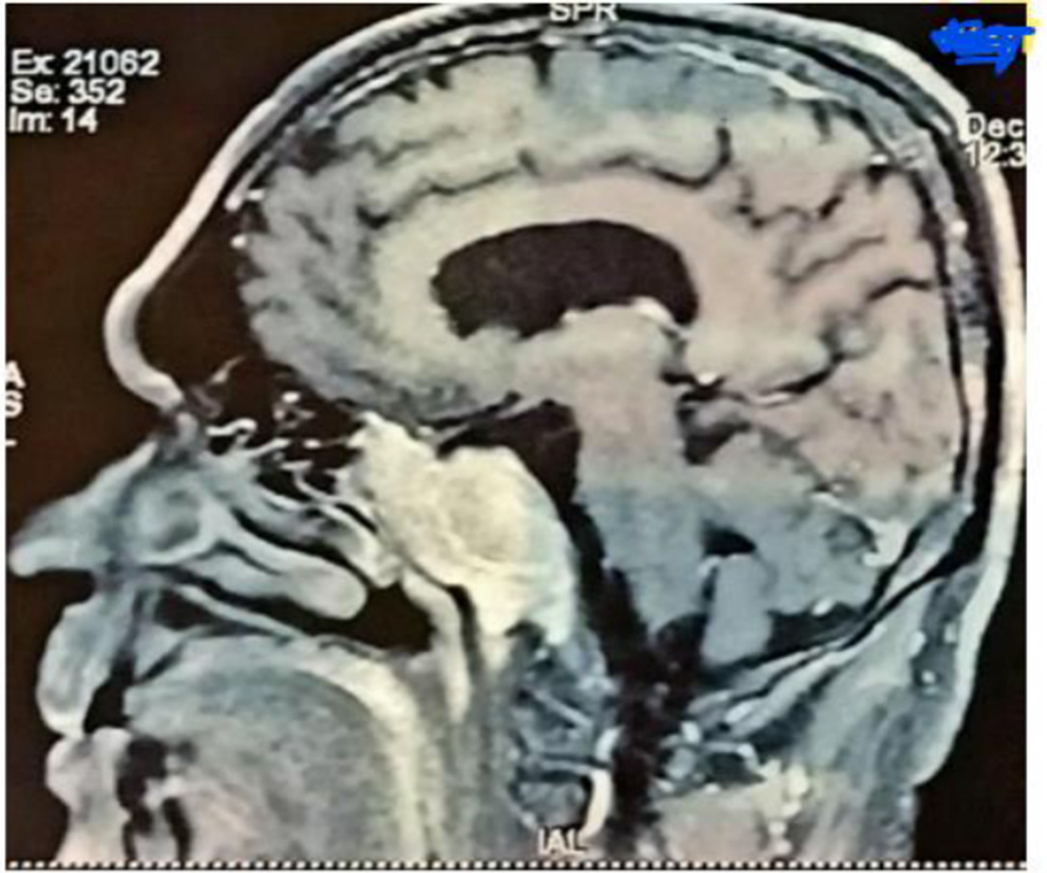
Brain magnetic resonance imaging (MRI) showing mass centered on clivus extending antero‐superiorly to the pituitary fossa, into bilateral sphenoid sinuses with their complete opacification and into bilateral ethmoid air cells and abutted bilateral optic nerves but had normal optic chiasma
